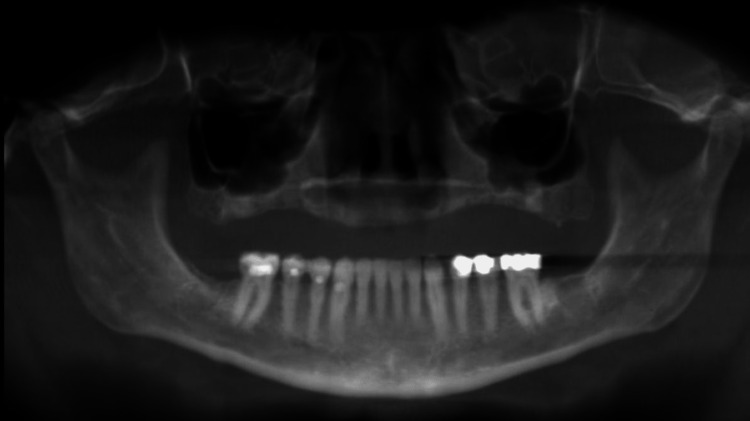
CBCT-reconstructed panoramic by Software A
